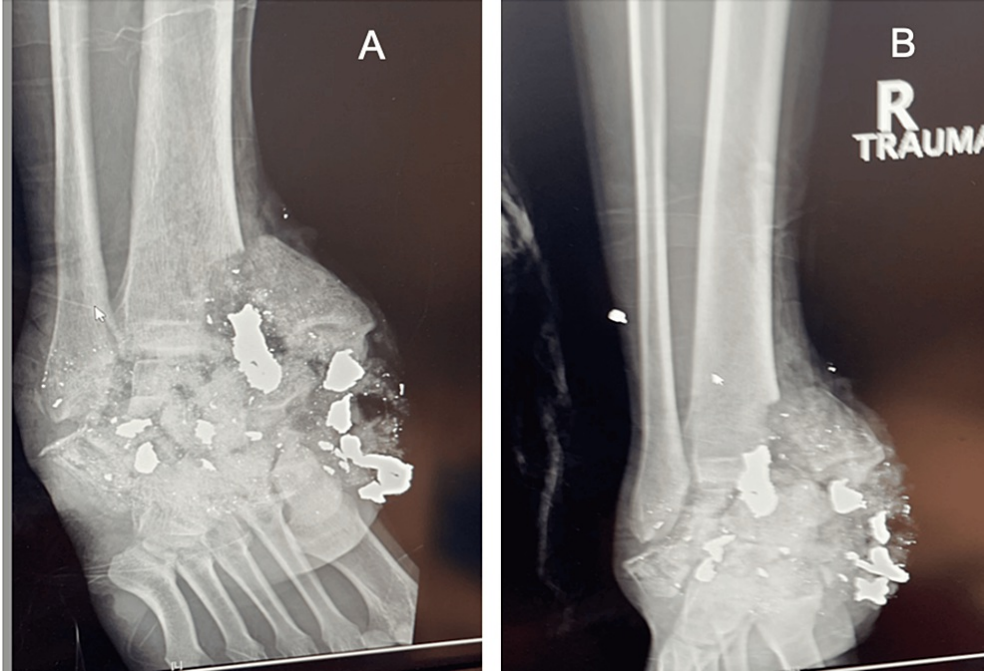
Preoperative radiographs of the injury. A: Oblique X-ray of the shotgun injury. B: Anterior-posterior X-ray from the shotgun injury.
